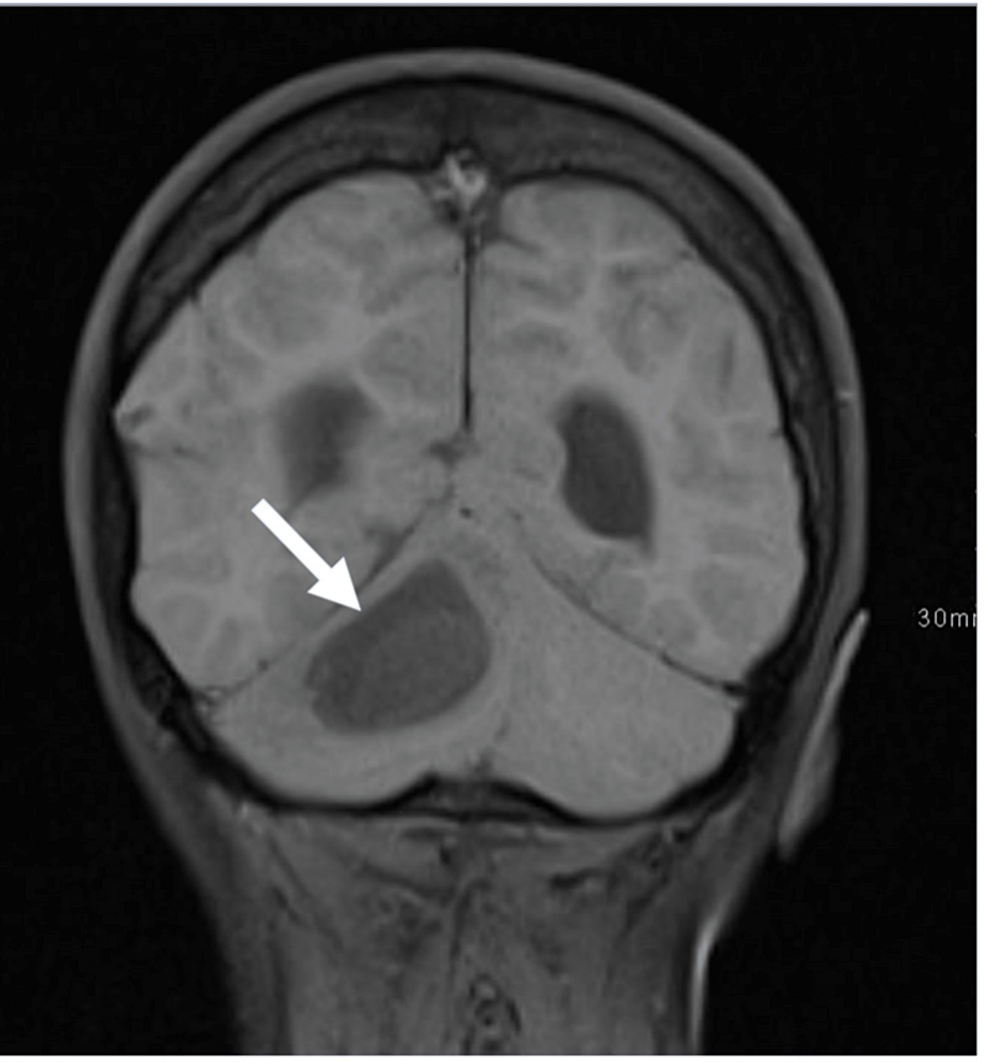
Coronal T1WI showing the heterogeneous low-signal intensity of the cyst (short arrow) in the right cerebellar hemisphere. T1WI, T1-weighted image
